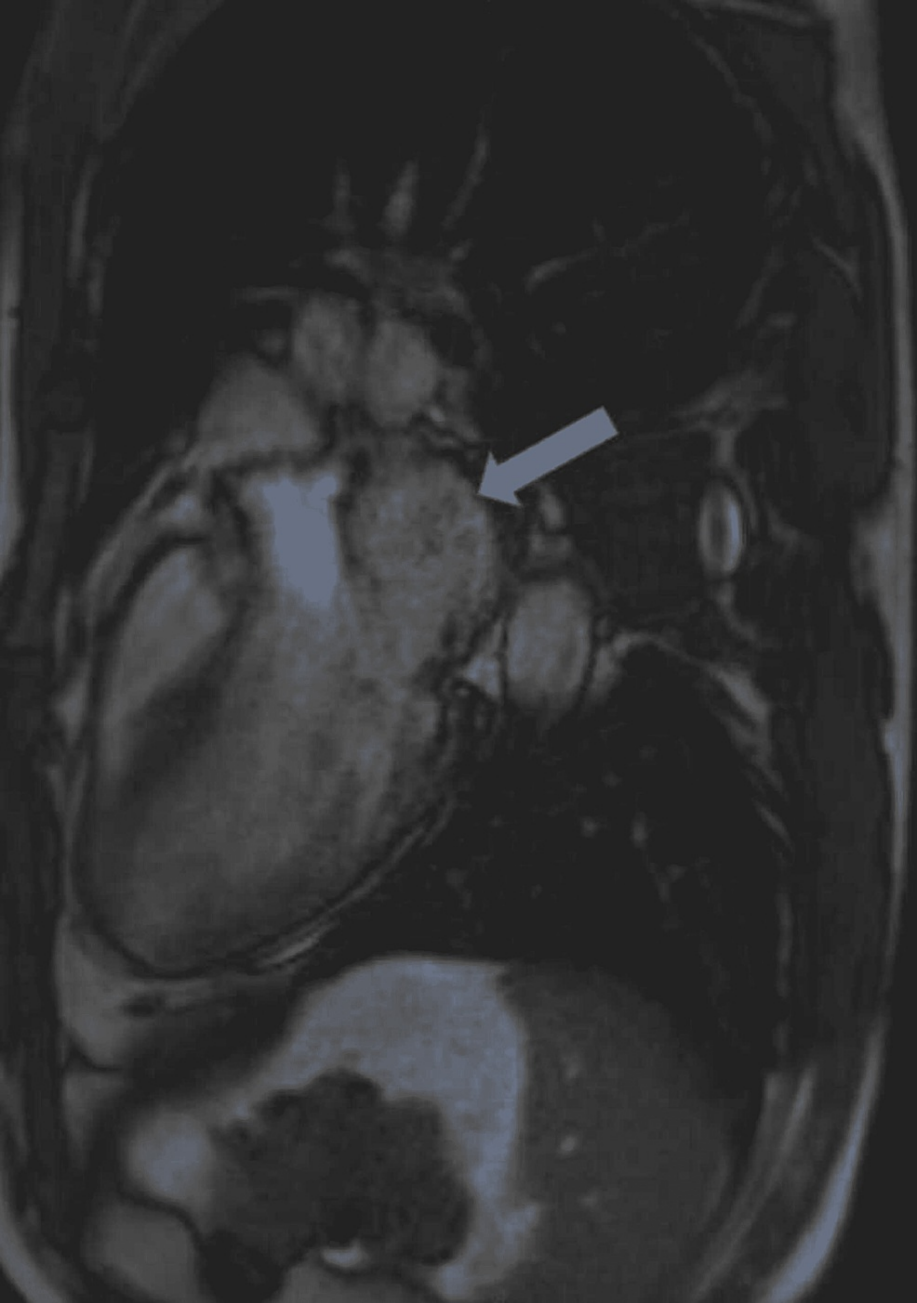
Cardiac magnetic resonance imaging shows left ventricular non-compaction cardiomyopathy (arrow).
